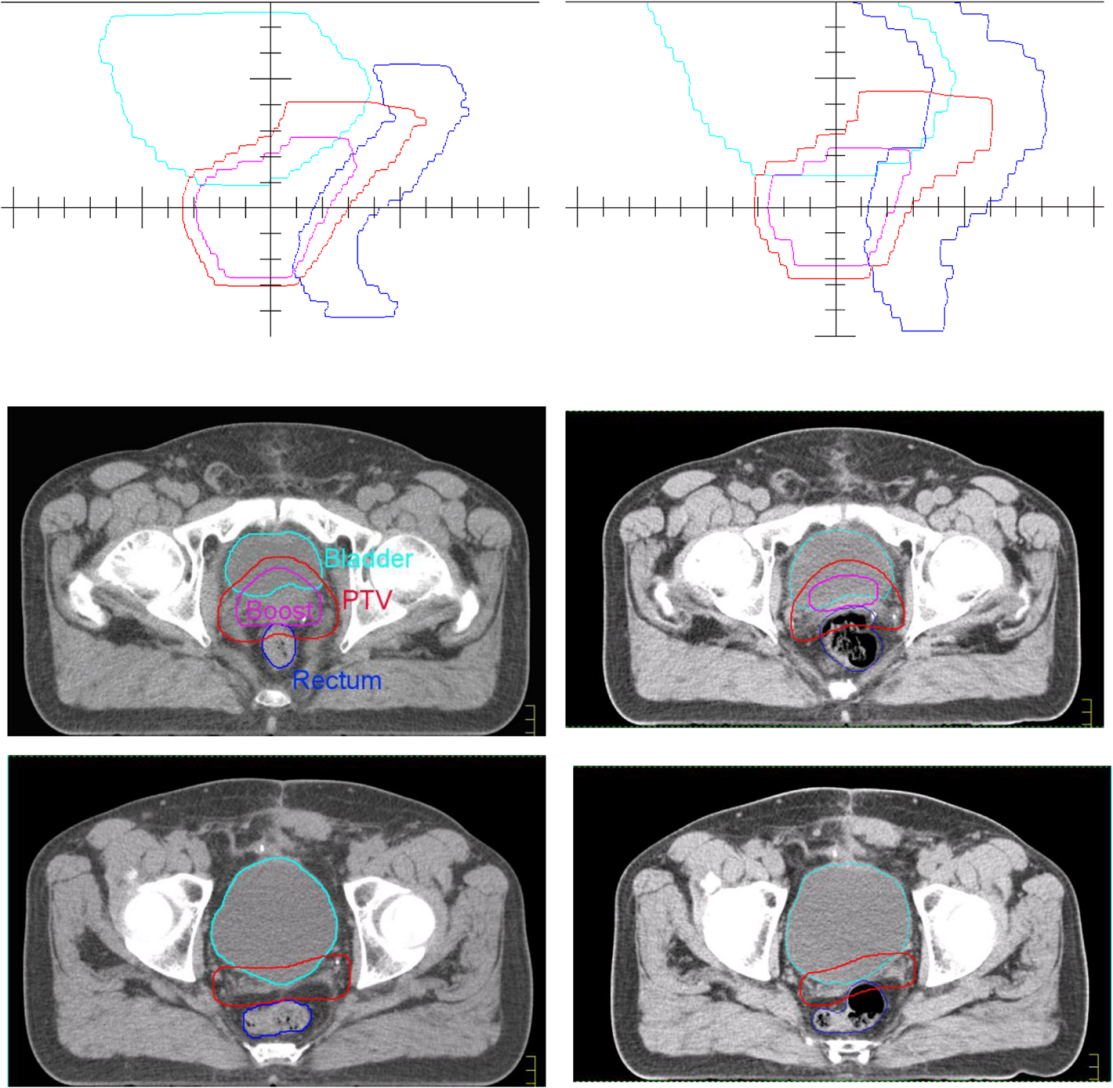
The prostate case of the largest volumetric difference between target SOIs in CT1 (left) and CT2 (right): top – BEVs; middle, bottom – CT layers showing an effect of increased bladder and rectum filling on Boost and PTV contours.
